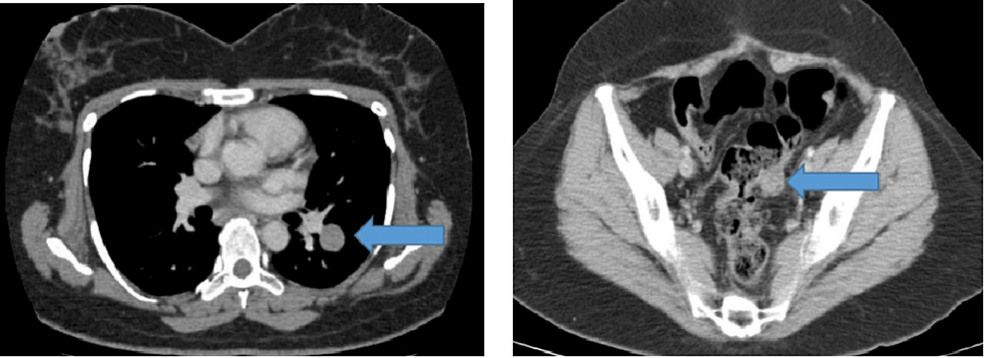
CT of the chest, abdomen, and pelvis (CAP) with contrast (axial views): disease progression by virtue of an increase in the size of left pulmonary and sigmoid colon nodules.
